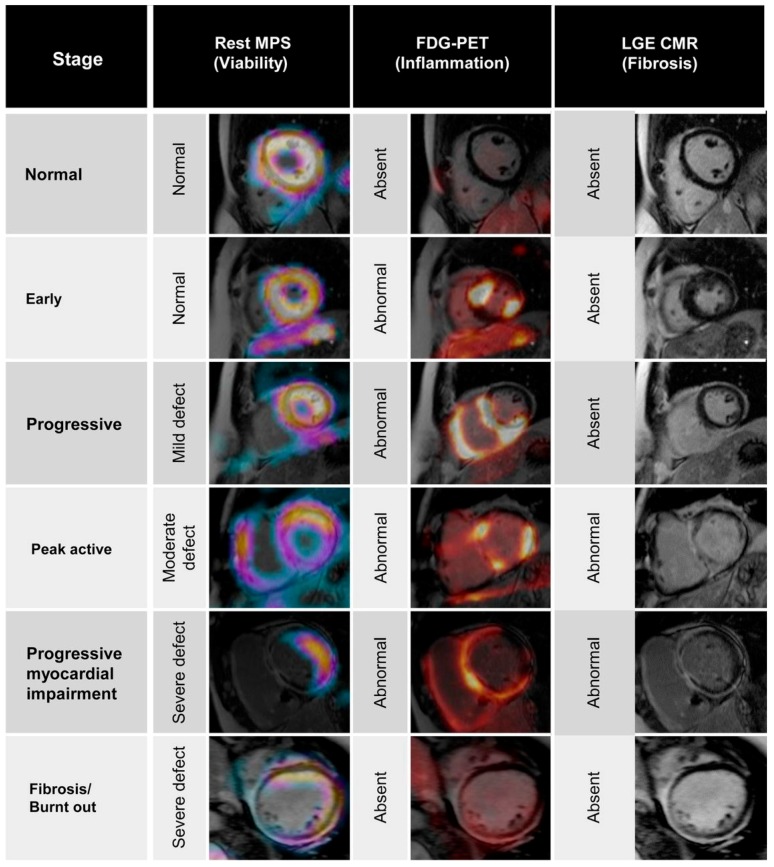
Staging of cardiac sarcoidosis using resting myocardial perfusion scintigraphy (MPS), FDG-PET and LGE-CMR. MPS shows increasing size of scarring as the disease advances. FDG-PET shows a heterogeneous pattern of inflammation in the intermediate stages, with exception to burnt-out stages in which there is no FDG uptake. LGE-CMR demonstrates the fibrotic changes, which is more prevalent in late stage sarcoidosis. Reproduced by Kouranos et al., 2015, by permission of *Br. Med. Bull.* [139].
